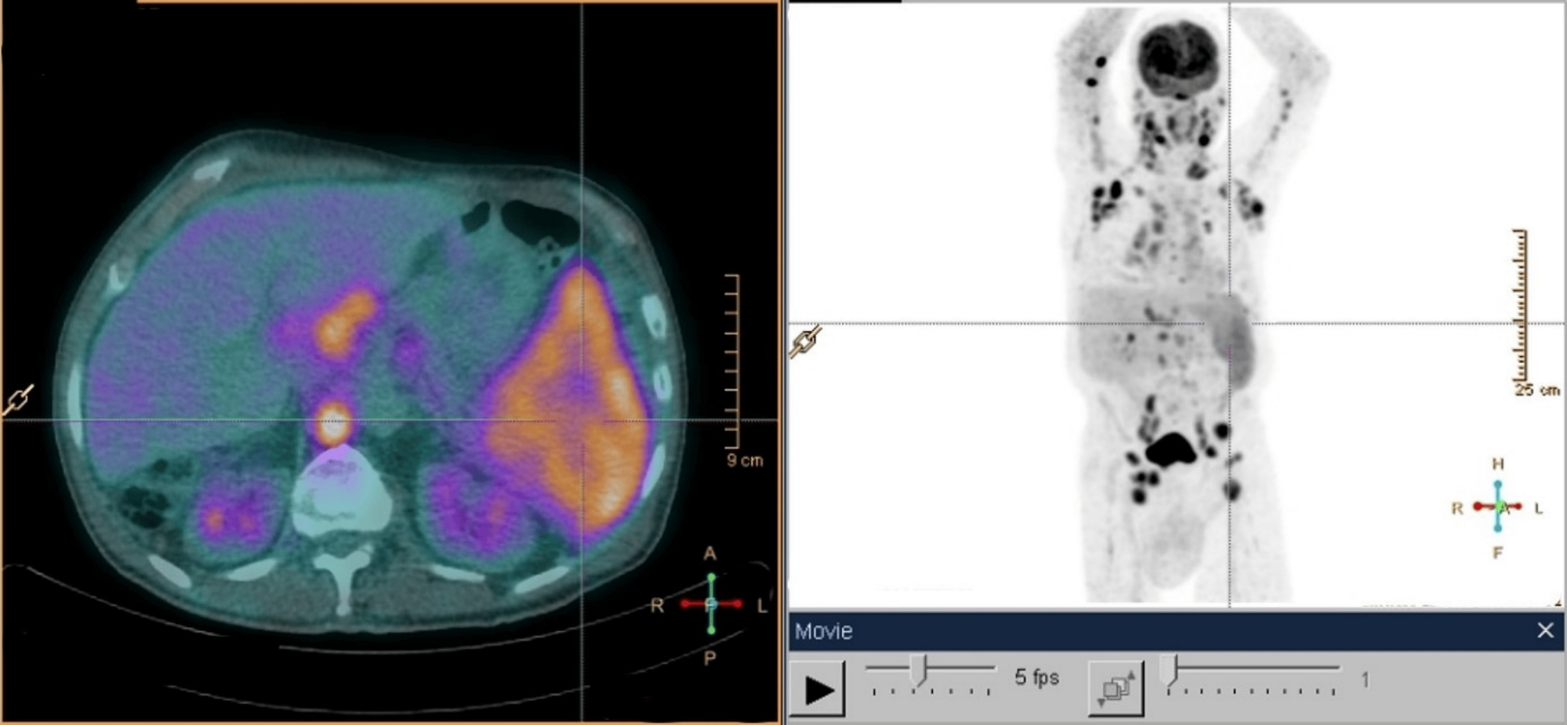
18F-FDG PET-CT PET-CT suggesting supra- and infra-diaphragmatic nodal, nasopharyngeal, and splenic involvement 18F-FDG PET-CT: 18F-fluorodeoxyglucose positron emission tomography-computed tomography
